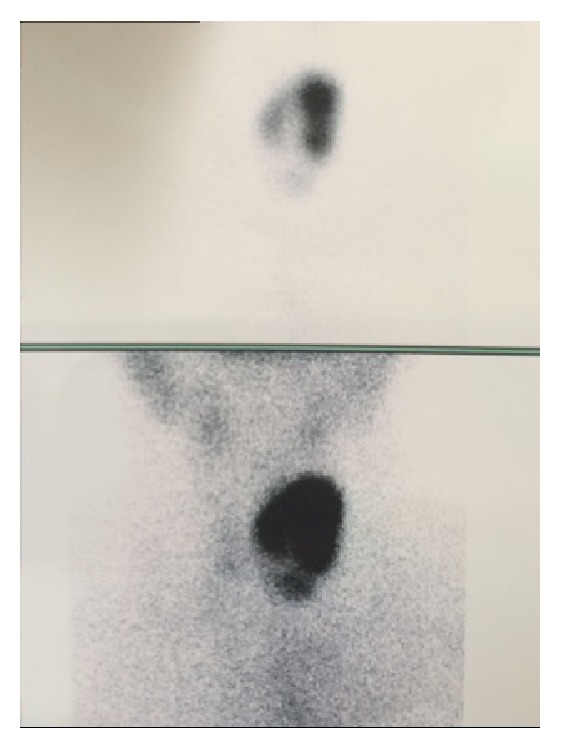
Thyroid technetium 99m scintigraphy showing hyperactive nodule on the left lobe and full suppression in the remainder of the gland.
